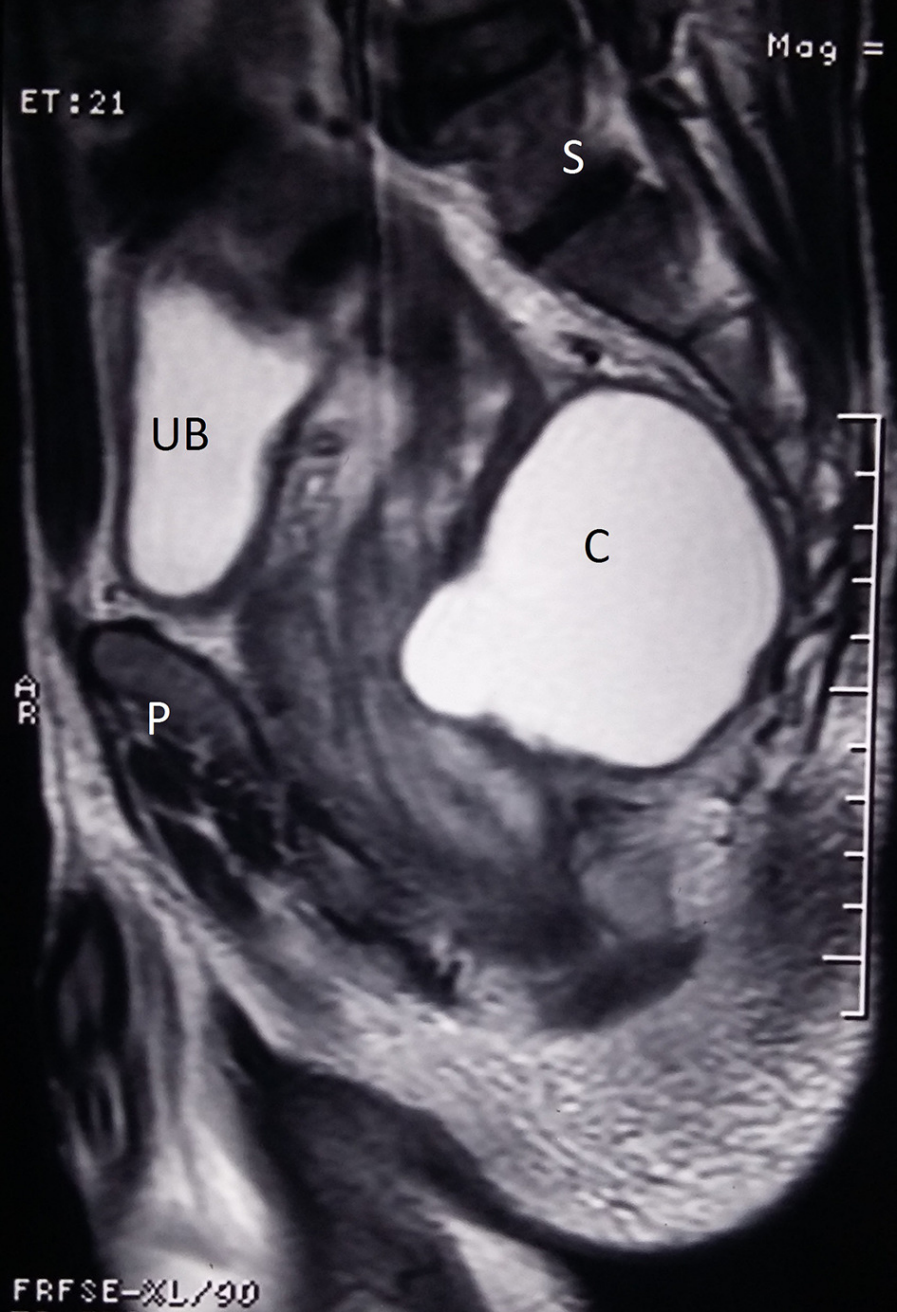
MRI sag: axial and sagittal *T*_2_ weighted images show the septa and a mural nodule within. The cyst wall appears to be tightly adherent to the posterior wall of the rectum (R). Urinary bladder (UB) with catheter bulb (B) in situ noted. S, sacrum; P, pubic bone.
